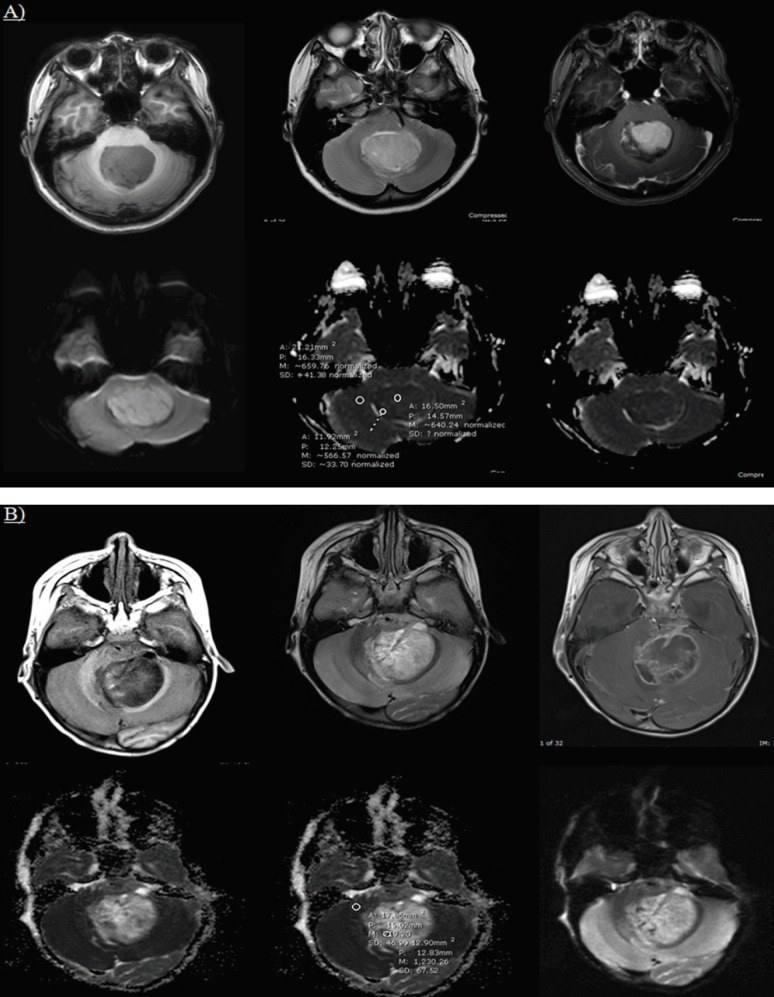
ADC Map Images of Medulloblastoma and Ependymoma Cases who had Overlapped Conventional MRI Findings by Using the ADC Ratio Cut-Off Level <1.18 for Tumor Differentiation. A, Medulloblastoma with T1W signal intensity score 3 (isointensity), ADC ratio was 0.92 and score 5 of DWI visual scale; B, Ependymoma with with T1W signal intensity score 3 (isointensity), ADC ratio was 1.87 and score 5 of DWI visual scale
